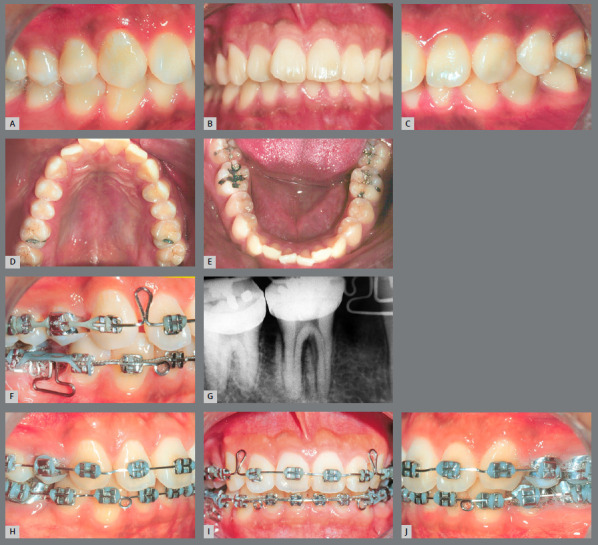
A-E) Class II division 1, subdivision with lower midline shift to the right. F-J) Treatment involved extraction of the upper first premolars, the lower left first premolar, and the lower right second premolar. The latter was extracted to facilitate anchorage loss for molar mesialization. Mechanics included T-loops and intermaxillary elastics for bodily molar movement (confirmed radiographically). However, mesialization of the lower first molar proved difficult, suggesting that avoiding the second premolar extraction on the lower right side could have allowed case completion with molars in Class II on that side.
